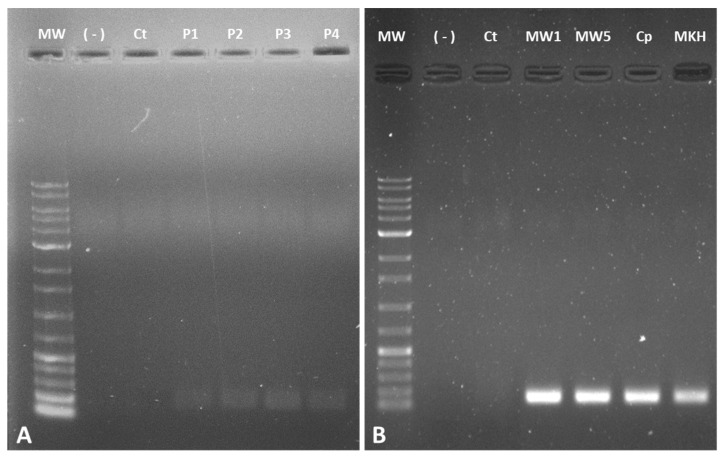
Analysis of GFP gene expression by RT-PCR after transfection of HeLa cells with (A) P1—PEI–DQA (10kDa)/TAT/pGFP, N/P of 20:2:1; P2—PEI–DQA (10 kDa)/TAT/pGFP, N/P of 50:2:1; P3—PEI–DQA (25kDa)/TAT/pGFP, N/P of 20:2:1; P4—PEI–DQA (25 kDa)/TAT/pGFP, N/P of 50:2:1; (B) MW1—MTS-WRAP1/pGFP, N/P of 5:1; MW5—MTS-WRAP5/pGFP, N/P of 5:1; Cp—CpMTP/pGFP, N/P of 5:1 and MKH—MTS-(KH)_9_/pGFP, N/P of 5:1. MW—molecular weight; (-)—PCR control; CT—non-transfected cells.
